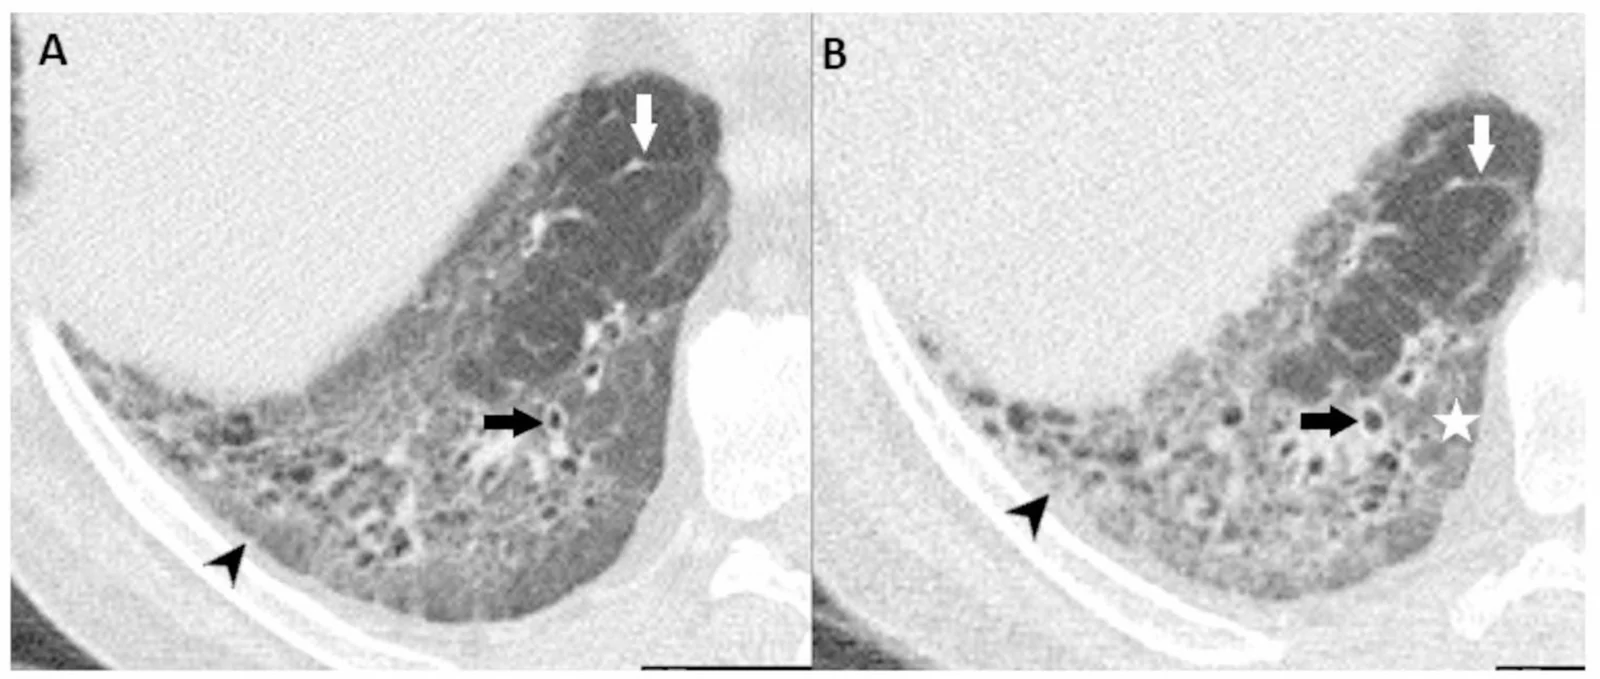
a and b: In a 70-year-old male patient with known rheumatoid arthritis, a fibrosing NSIP pattern is observed in high-resolution computed tomography scans taken at 7-year intervals. In axial sections taken from the lung bases, irregularities are observed to become evident in the subpleural area consistent with fibrotic changes (black arrowheads). Interlobular septal lines are seen as tiny lines outlining secondary pulmonary lobules forming polygonal shapes in both scans (white arrows). They are more prominent in the later section in figure b due to progressive fibrosis. In consecutive sections, an increase in ground- glass opacities (asterix) are visible, as well as traction bronchiectasis (black arrows)
